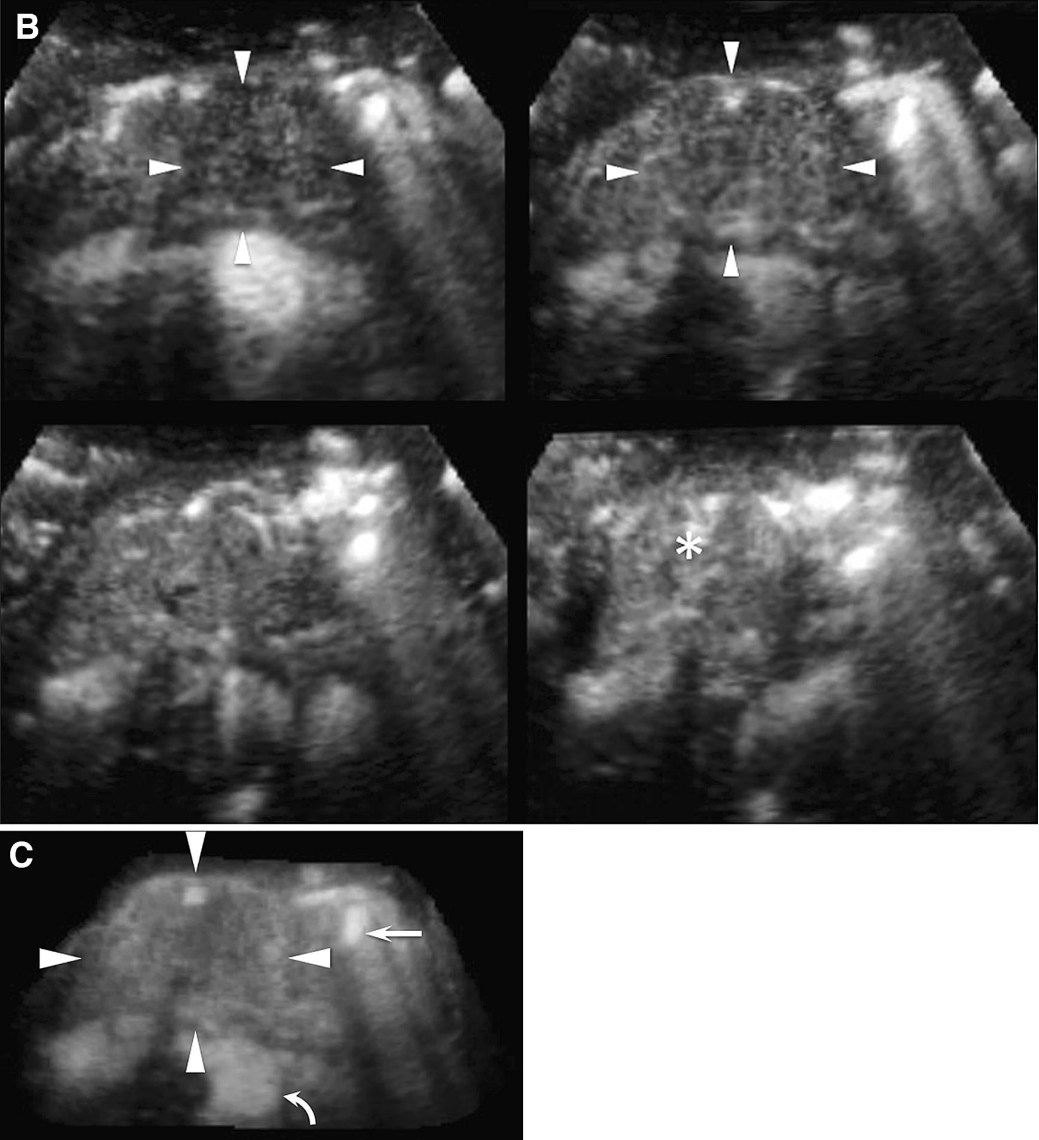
Conventional 3D US and CE 3D US images acquired in the transverse plane of a 78-year-old woman with MFP (maximum diameter, 33 mm) in the pancreatic head. A TUI of 3D US (plane A) in the B-mode shows a hypoechoic lesion in the pancreatic head (*arrowheads*). The image on the lower right shows the pancreatic parenchyma in the pancreatic body (*asterisk*). B TUIs of CE 3D US (plane A) in the arterial phase show an isovascular lesion (*arrowheads*) compared with the pancreatic parenchyma (*asterisk*). Dense fine vessels are seen in a whole lesion, hypoechoic on B-mode US, similar to the pancreatic parenchyma. C Sonographic angiogram in the arterial phase, rendered in the average intensity mode, shows homogeneous enhancement (*arrowheads*) of both the pancreatic head and body. Superior mesenteric artery (*arrow*) and abdominal aorta (*curved arrow*) appear around pancreas.
